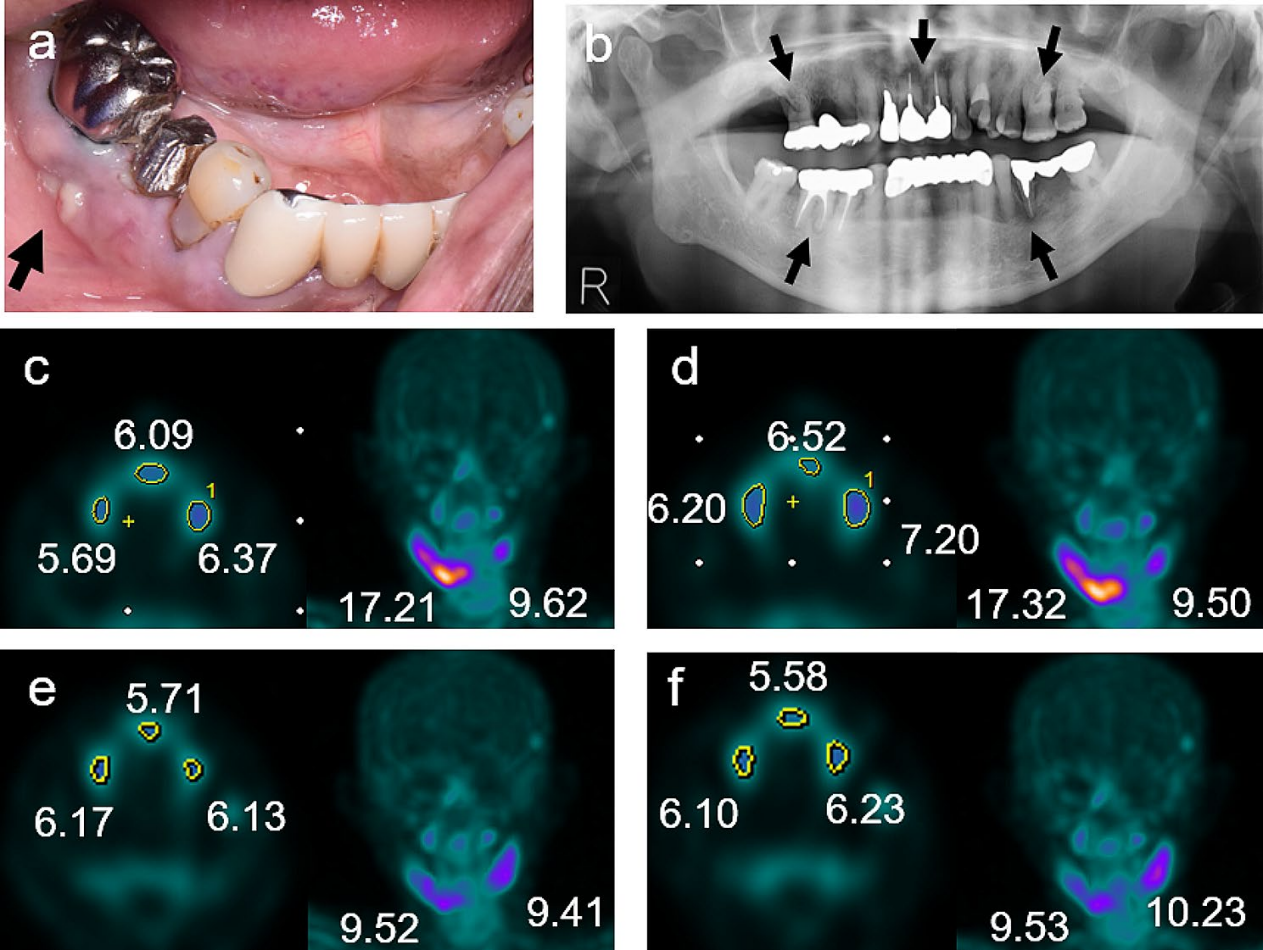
Depiction of a representative case (Patient 1). Patient 1 was a 75-year-old man who had been treated for multiple myeloma at our hospital. Zoledronate was administered four times, and denosumab was administered nine times. Swelling and drainage of pus from the fistula in the buccal gingiva of the second premolar of the right mandible (black arrow) (a). In the panoramic radiograph, periapical lesions are seen in five teeth (16, 21, 26, 35, and 45) (black arrows) (b). In the 2-h image from the first SPECT, strong accumulation in the right mandible, moderate accumulation in the left mandible, and weak accumulation in the maxilla are observed at three sites (c). There is an equivalence between the findings of the 3-h and 2-h images for the first SPECT (d). In the 2-h image for the second SPECT (conducted 3 months later), the strong accumulation in the right mandible has improved to moderate accumulation (e). There is an equivalence between the 3-h and 2-h images for the second SPECT (f). *SPECT*, single-photon emission computed tomography
